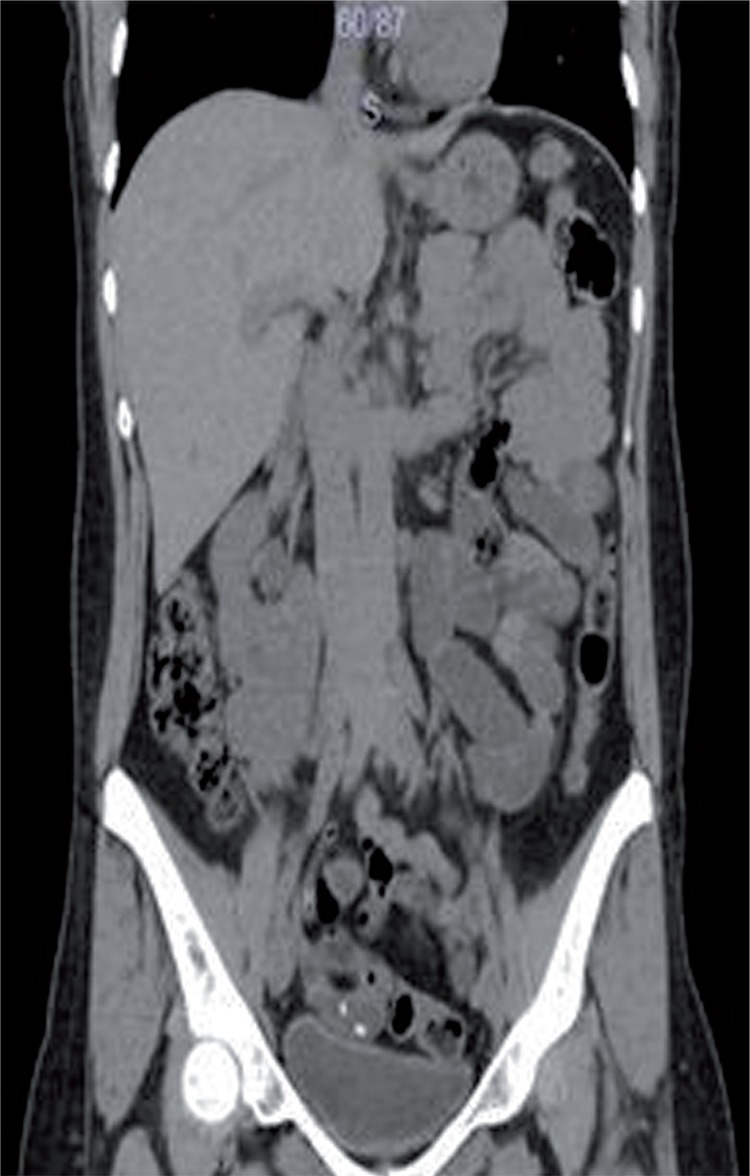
Computed tomography scan of right kidney.
